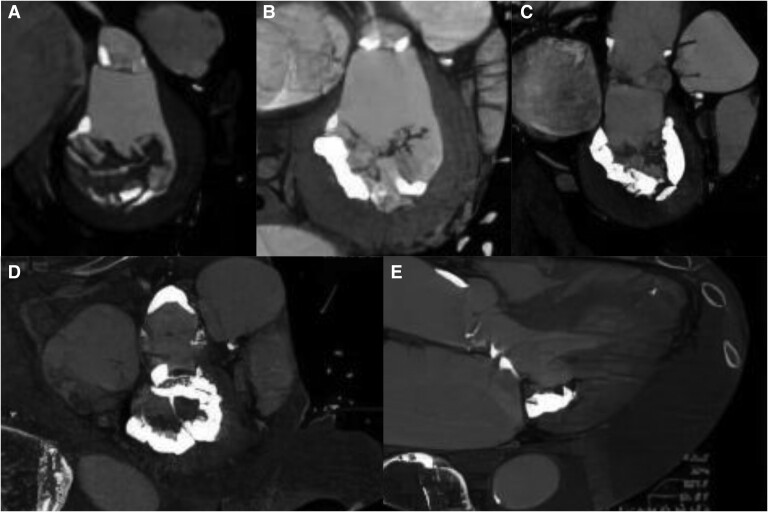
Qualitative evaluation of mitral valve calcification assessed using multi-detector CT. MAC severity was determined according to the visual circumferential involvement of the mitral annulus as follows: (*A*) mild (less than one-third of the annulus), (*B*) moderate (between one-third and one-half of the annulus), and (*C*) severe (more than half of the annulus). Valve leaflet calcification was identified by observing the mitral valve in two directions, the short axis (*D*) and long axis (*E*), to distinguish it from LV outflow tract calcification or MAC.
